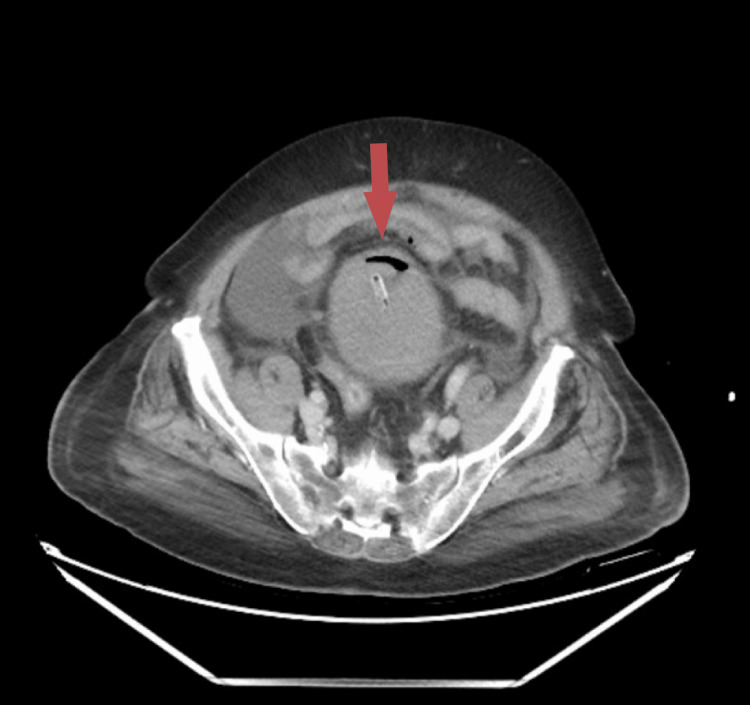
CT of the kidney, ureter, and bladder (axial view) showing emphysematous cystitis complicated with bladder rupture and large high density intra-abdominal collection
